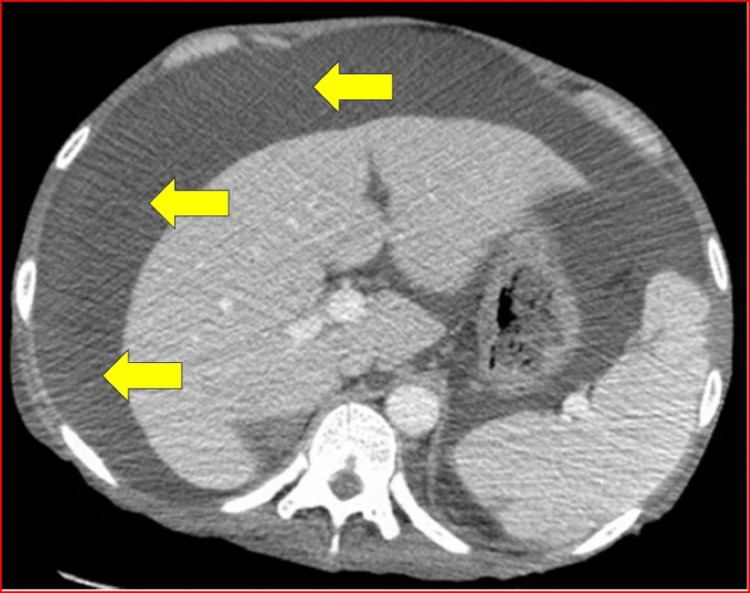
CT abdomen with ascites (yellow arrows).
